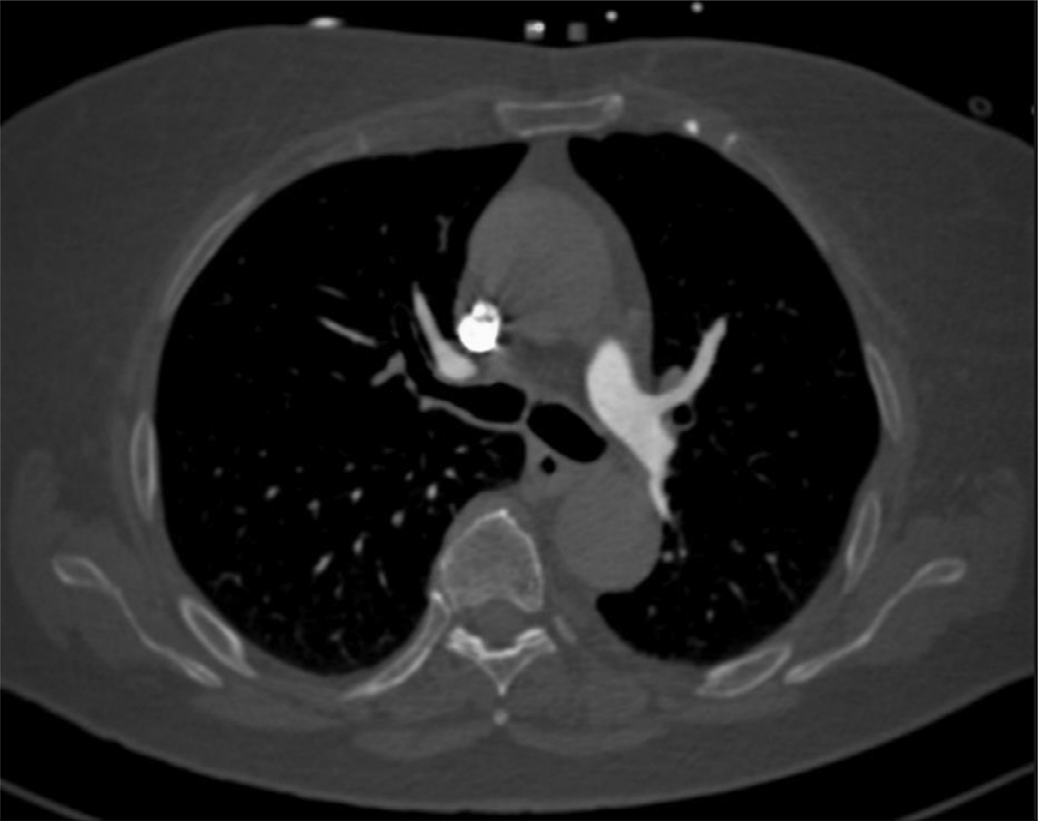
pulmonary arterial phase, Chest CT. Complete vessel recanalization, perfusion defects are no longer noticeable.
